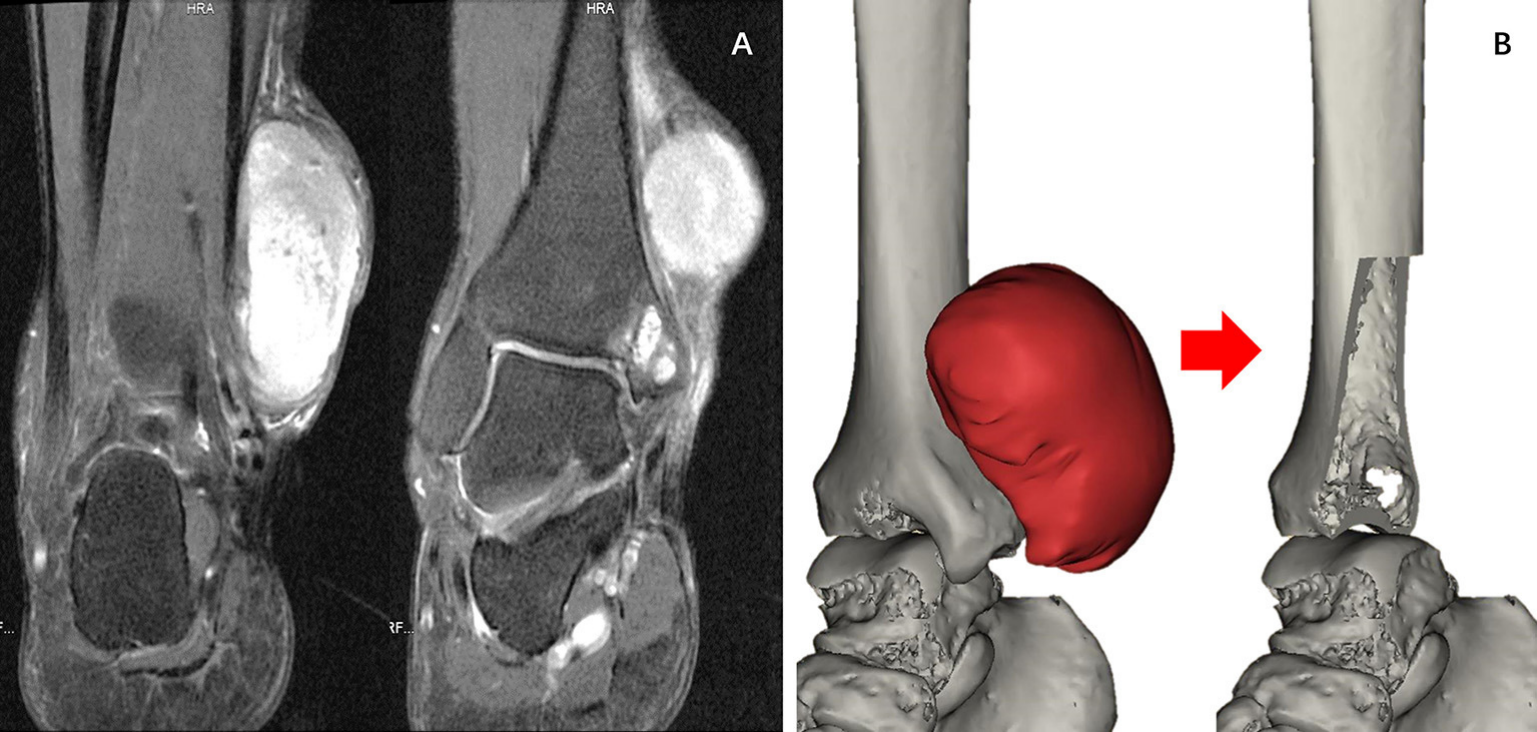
(A) Magnetic resonance imaging. Soft tissue tumors can be seen on the anterior medial side of the ankle joint, and the ankle joint is slightly involved. (B) Reconstructed 3D models of the extent of the resection and the size of the ankle joint defect.
